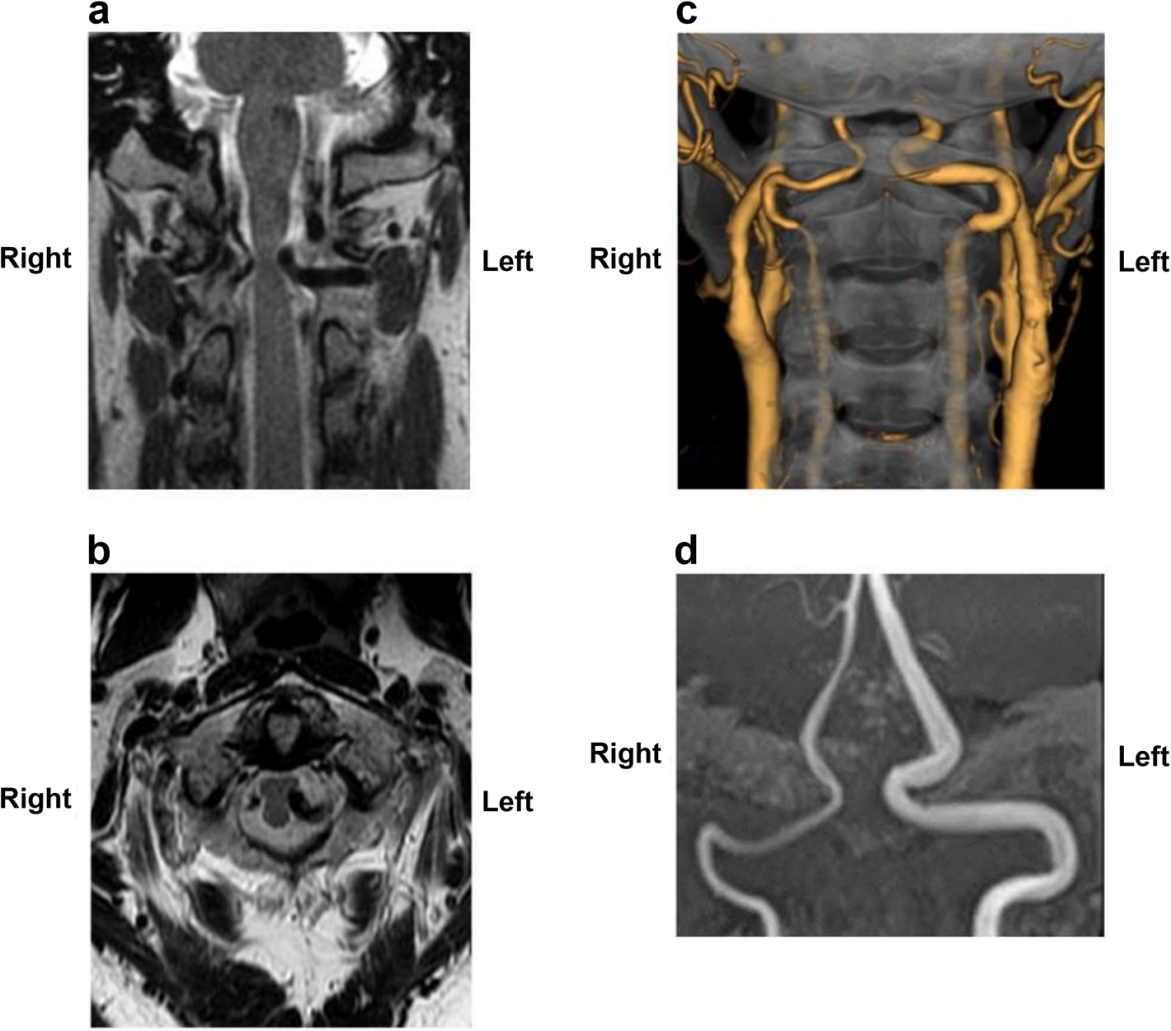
Preoperative radiological findings. Magnetic resonance image showing compression of the spinal cord caused by bilateral persistent first intersegmental artery (PFIA); the spinal cord was compressed strongly by the left PFIA (a, b). Both vertebral arteries pass through the foramen transversarium up to the C2 level and then branch as PFIAs on each side. Both PFIAs enter the spinal canal at the C1/C2 level and merge with each other at the distal side of the basilar artery (c, d). a: T2-weighted magnetic resonance image (coronal). b: T2-weighted magnetic resonance image (sagittal). c: Computed tomography angiography image. d: Magnetic resonance angiography image
